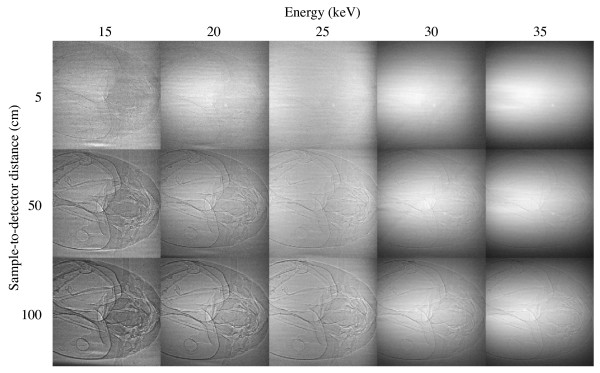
Video image quality as a function of x-ray energy and sample-detector distance. Data are from an ant head (*Camponotus pennsylvanicus*) using a Cohu 4950 video camera. Within each column, the absorbed x-ray dose on the insect is constant. For all images, the photon flux was kept at approximately 2 × 10^10 ^ph/s/mm^2^.
